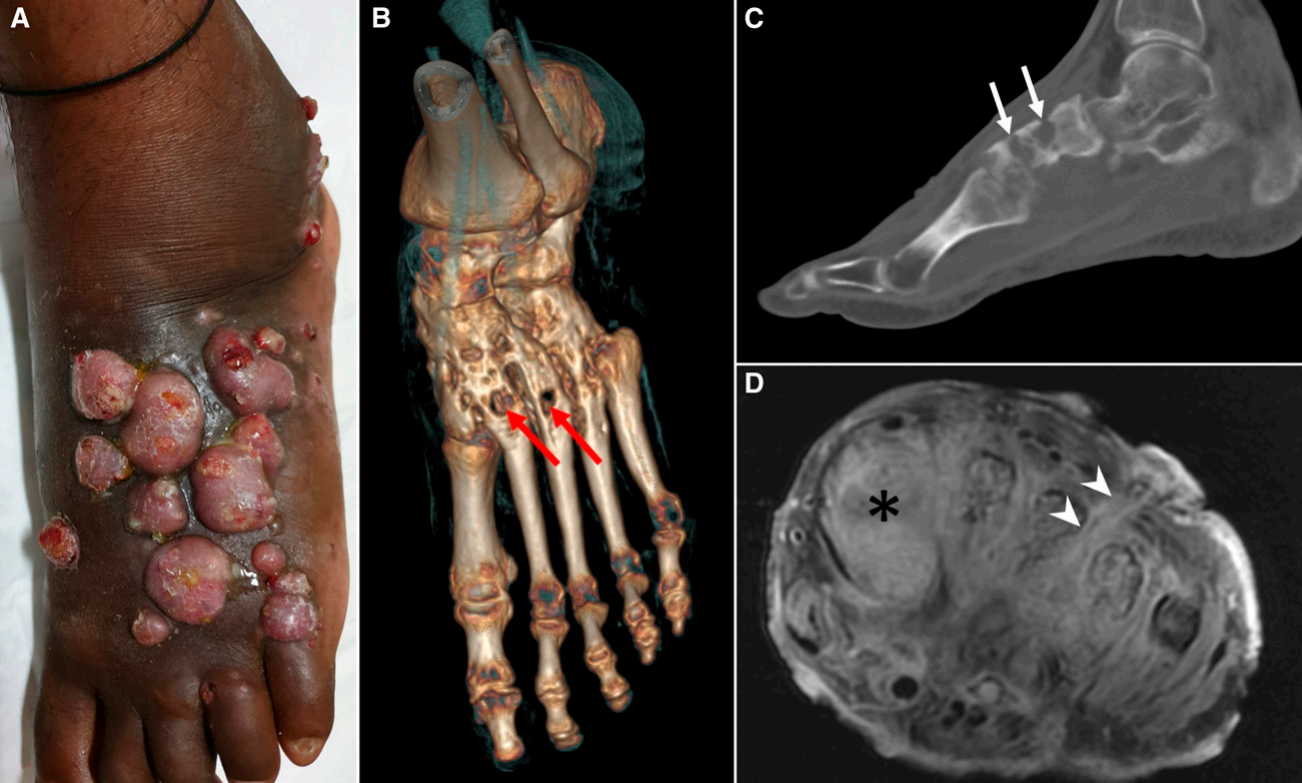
(A) Multiple nodular swellings with sinuses discharging serosanguineous pus over the left foot. Three-dimensional volume-rendered reformatted computed tomography (CT) image (B) and sagittal CT image (C) show multiple osteolytic lesions (arrows) in tarsal and metatarsal bones. Fat-suppressed contrast-enhanced T1-weighted magnetic resonance image (D) showing diffuse hyperintensity (asterisk) and enhancement involving the metatarsal bones and surrounding soft tissues. Note sinus tract denoted by arrowheads. This figure appears in color at www.ajtmh.org.
